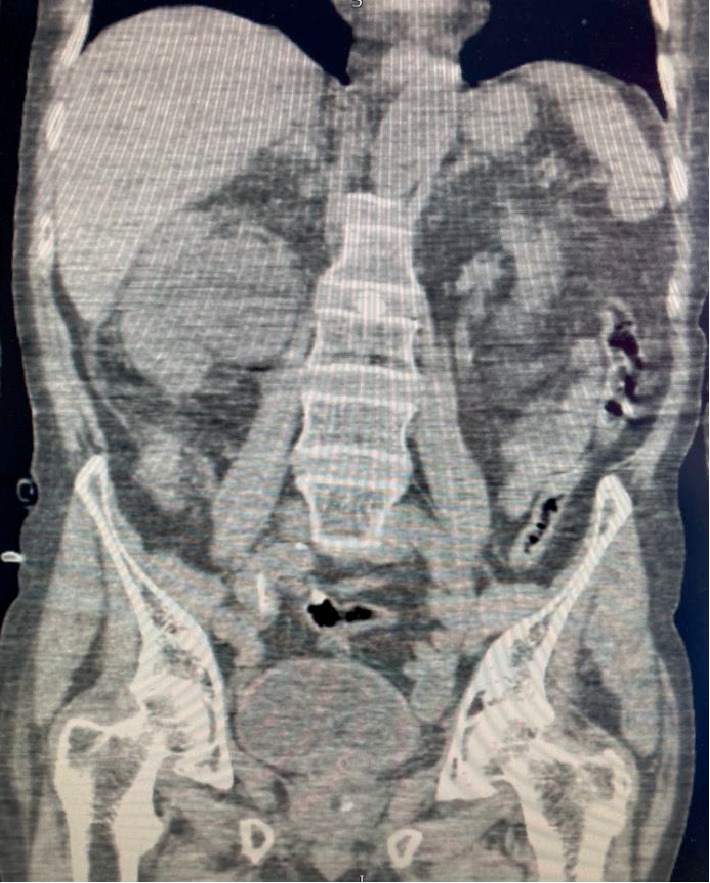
CT KUB showing significant right sided hydronephrosis along with perinephric fat stranding.
